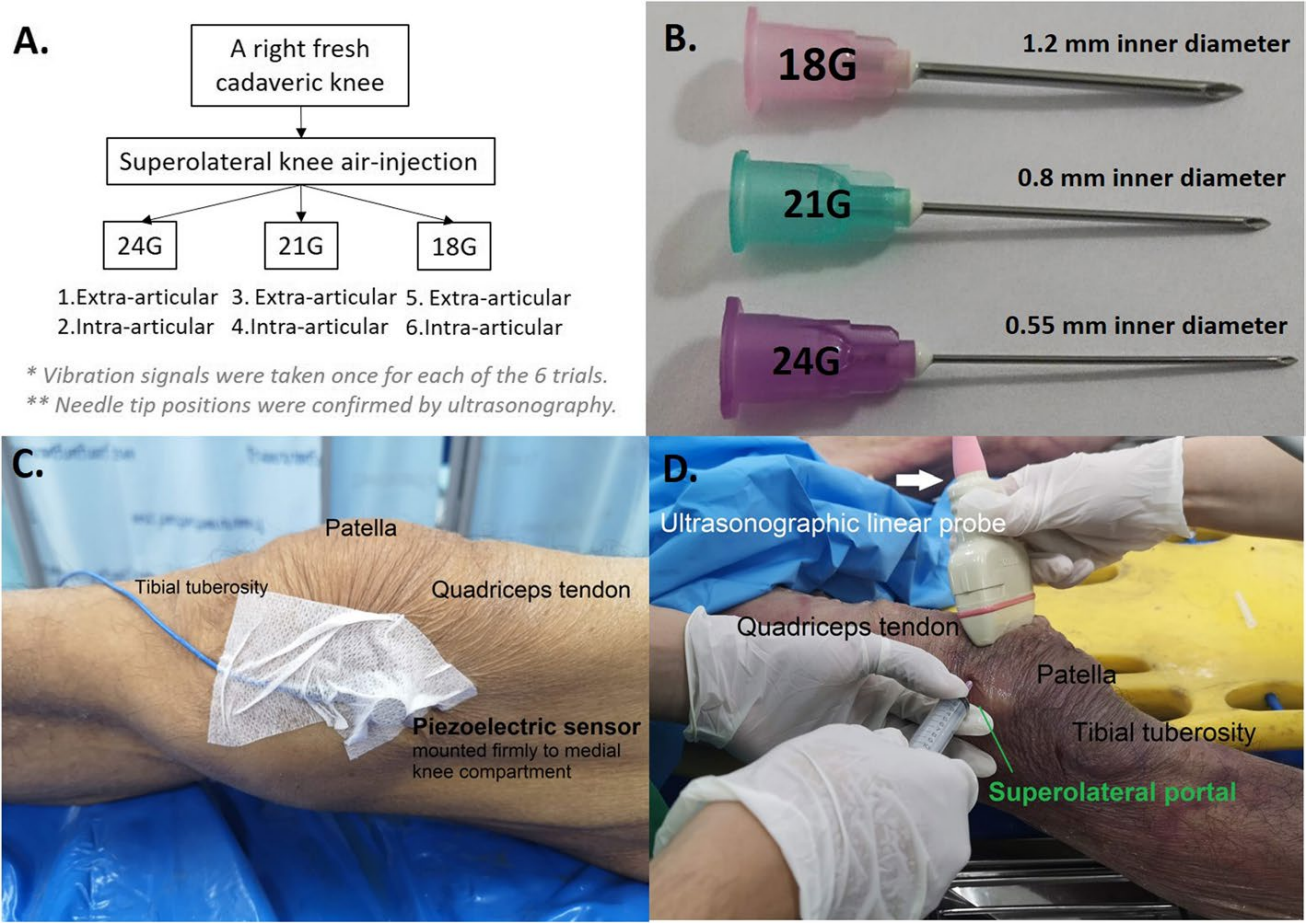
The workflow of the study consisted of vibration signals measurement for six consecutive trials of the knee injections (A). Three needle sizes were used (B). Experimental setup was done by mounting the accelerometer firmly on the medial collateral ligament just below the medial border of patella, about the mid-patellar level (C). An empty syringe was placed at supero-lateral knee, ready for the 2-ml air injection, and a linear ultrasound probe was positioned on the quadriceps tendon above the patella (D)
